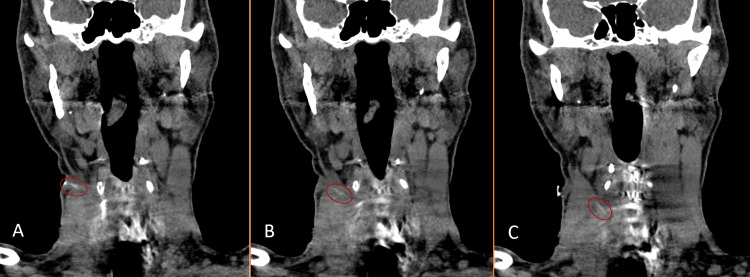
Neck CT with oral Gastrografin conforming the formation of an esophagocutaneous fistula and the absence of leak. (A-C) Sequential coronal views showing the presence of contrast (faint radio-dense line) in the esophagocutaneous fistula, which is indicated by the red circles.
